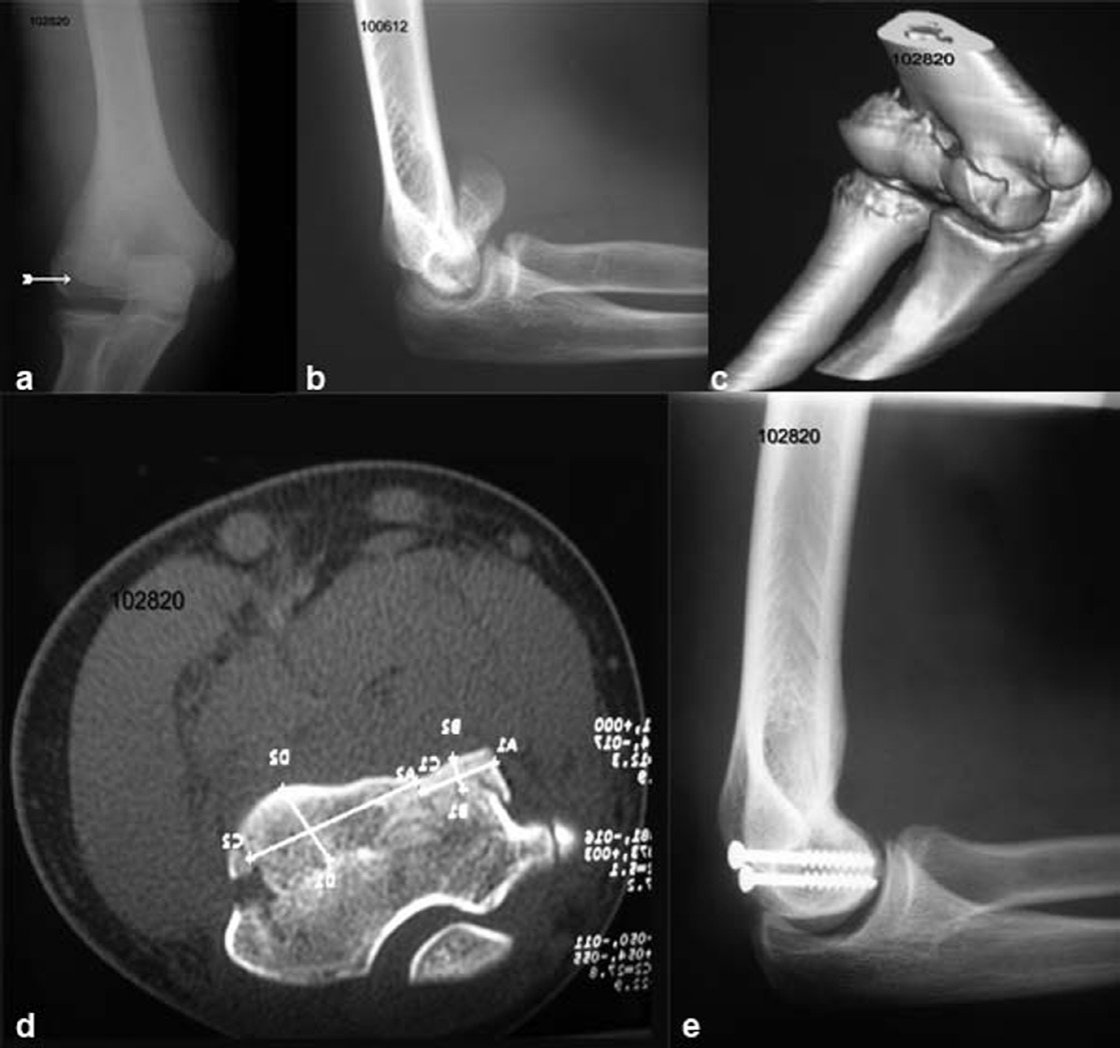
(a) Anteroposterior view of the elbow showing the fracture (white arrow) which is easily missed in emergency room. (b) True lateral view of elbow showing double arc sign. (c) 3D CT scan showing the involvement of entire trochlea. (d) Axial section of CT scan showing the measurements of the fragments. (e) Lateral X-ray of the elbow showing fixation with 4 mm cancellous screws
